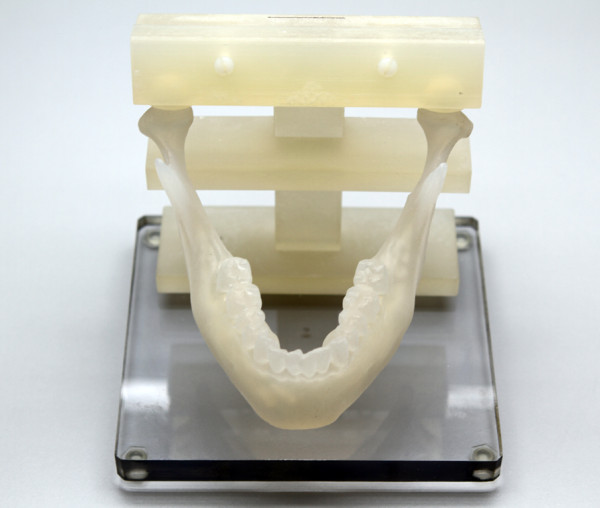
Scanning jig. Jig design for the CT scanning with the mandible in place.
